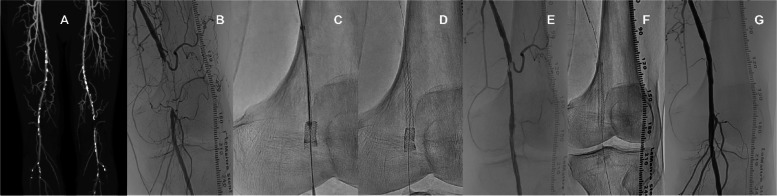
Process of endovascular therapy. A Computed tomography angiography image showing occlusion of the left superficial femoral artery distal to the proximal popliteal artery. B Basic angiography of the left limb. C Invagination occurred during stent deployment. D Implanted invaginated stent unavoidably. E Angiography for insufficient stent dilation and proximal thrombus clusters. F Another new IWS of the same size was accurately placed. G Final angiography after stent reimplantation
